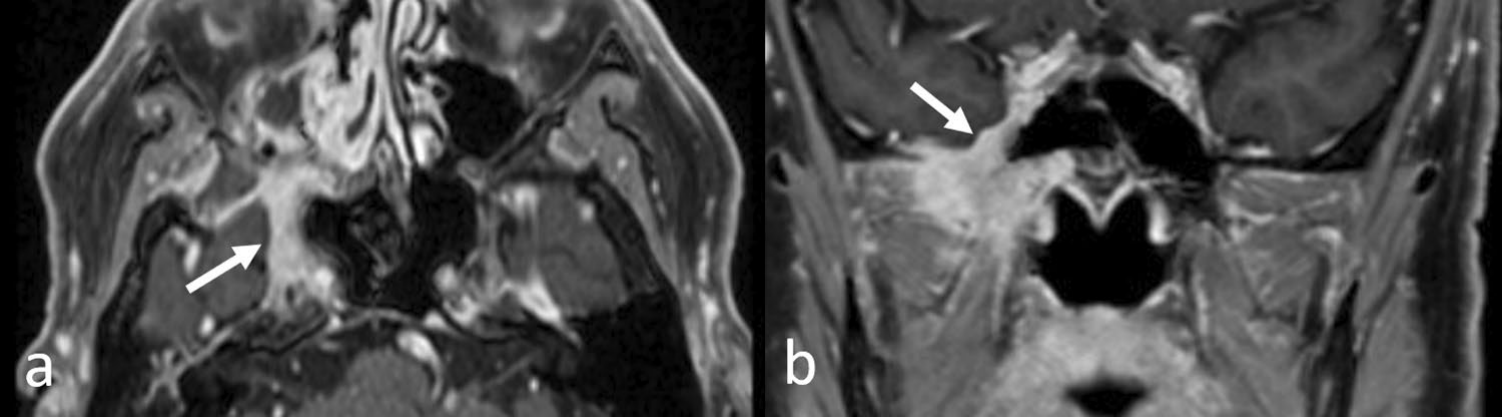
Perineural spread of cranial nerve V in a patient with a sphenoidal squamous cell carcinoma. Axial a and coronal b reconstructed contrast-enhanced 3D fat-suppressed T1-weighted images show enlarged and enhanced cranial nerve V with its characteristic location (arrows)
